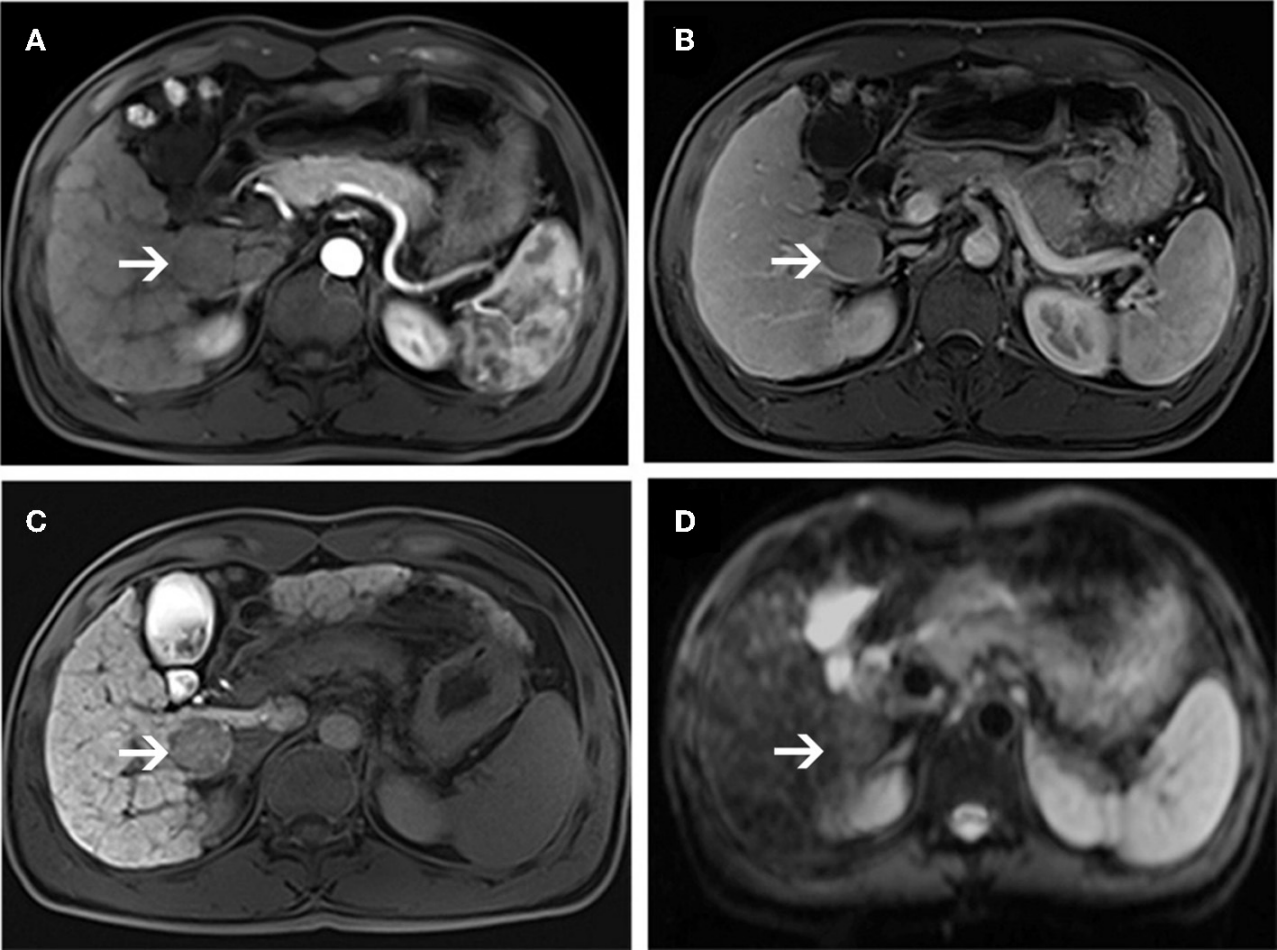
MR images of a 66-year-old woman with a pathologically proven HCC (white arrows) and a history of hepatitis C virus infection. An arterial-phase image (A) shows a hypovascular nodule in segment I of the liver. An equilibrium phase MR image (B) shows a slightly hypointensity nodule compared to the surrounding liver parenchyma. A hepatobiliary phase image (C) shows a hypointensity lesion compared to the surrounding liver parenchyma. On diffusion-weighted image (D), the lesion is nearly isointensity compared to the surrounding liver parenchyma.
